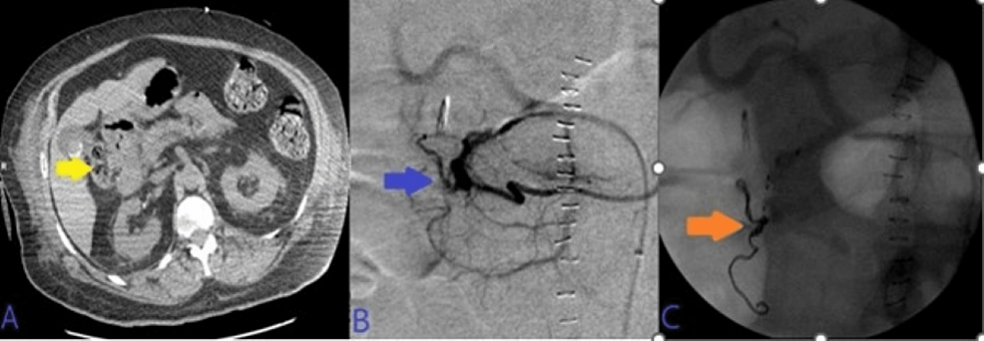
A: CT of the abdomen demonstrated dense-fluid-filled duodenal wall thickening and ulceration crater as seen on endoscopy. B: Super-selective GDA angiograms demonstrated many focal bleeding blush adjacent to endoscopic clip. C: Post-embolization celiac angiogram demonstrated no evidence of active bleeding signs, and complete resolution of the above findings after deployment of one coil and two vascular plugs in appropriate positions along the GDA. CT: computed tomography; GDA: gastroduodenal artery.
